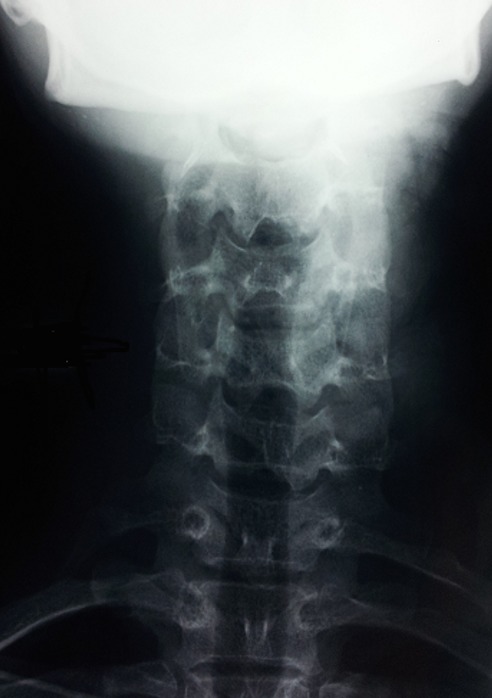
Radiographie standard de face
